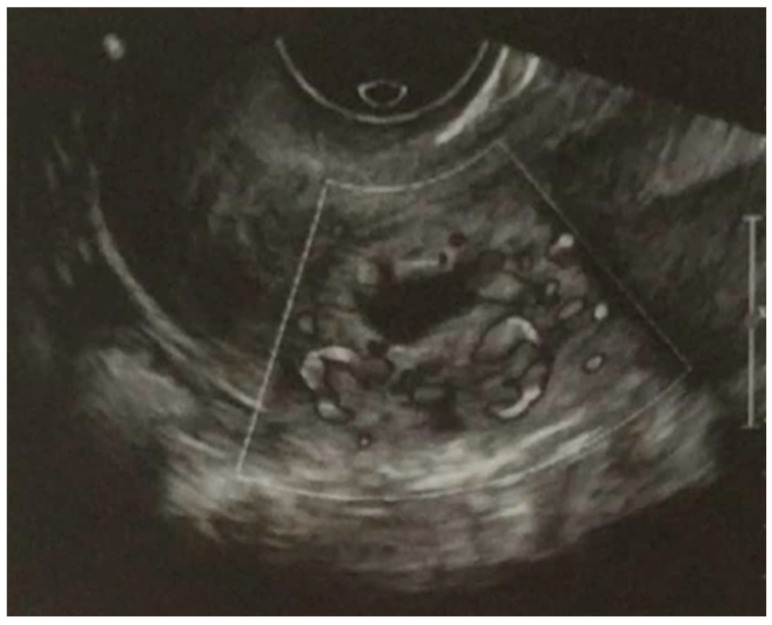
Ultrasound image before the start of treatment.
